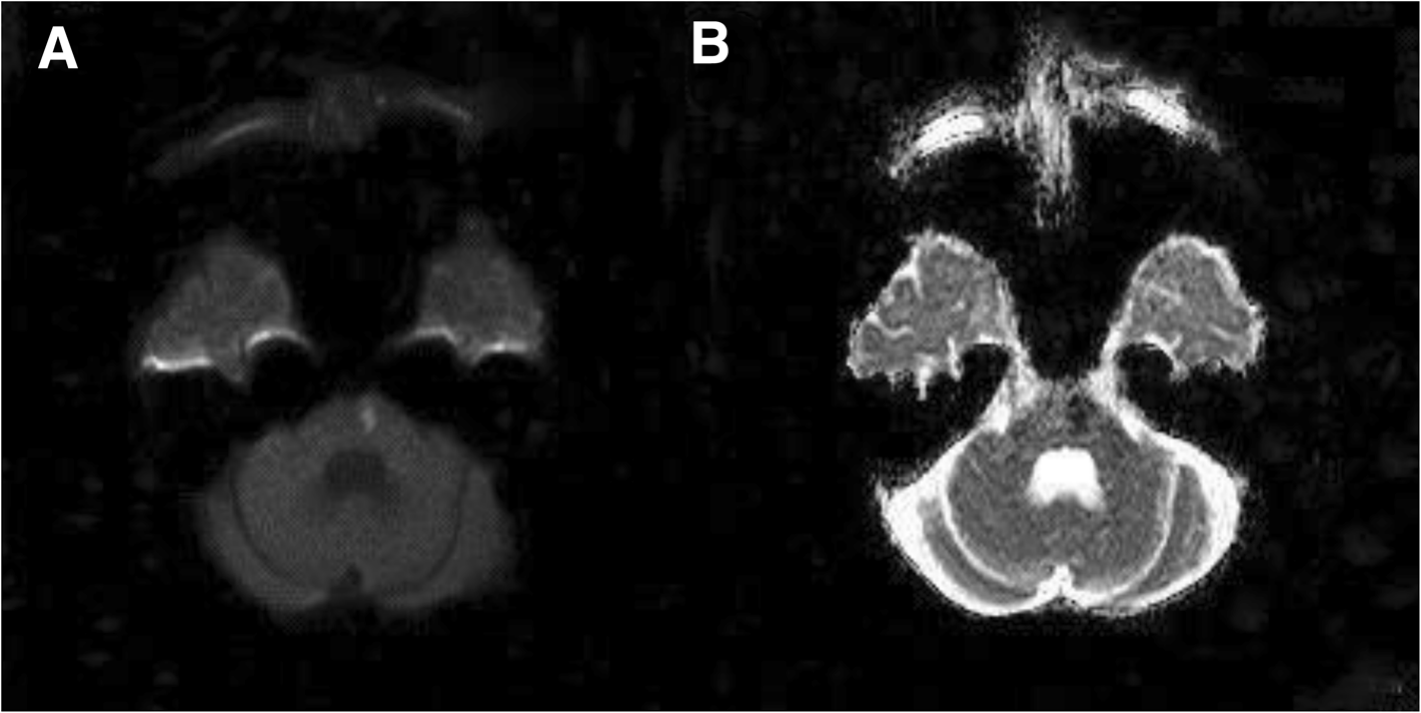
Brain MRI of the patient. Diffusion weighted imaging (a) and apparent diffusion coefficient image (b) showing acute infarction in the left anterior paramedian of the mid-pons
